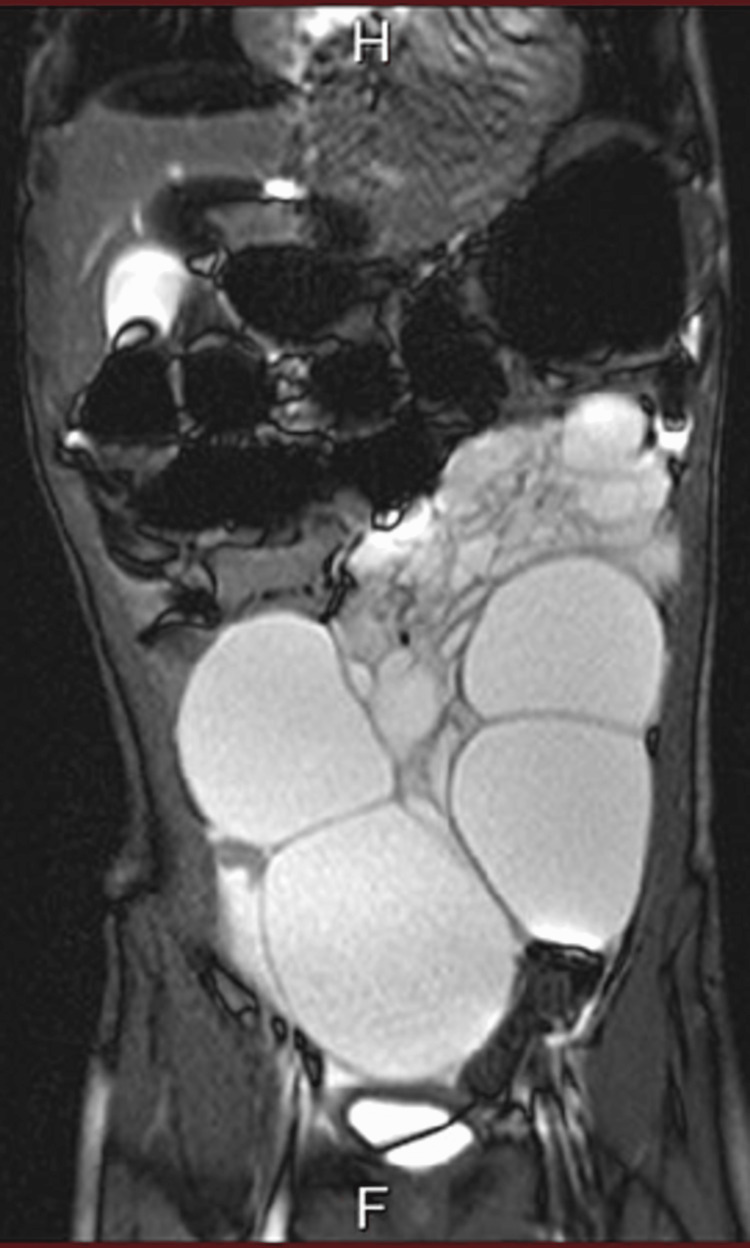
Coronal MRI of the abdomen shows cystic lesions filling the abdominal cavity and displacing the small bowel to the right upper quadrant. MRI: Magnetic resonance imaging
